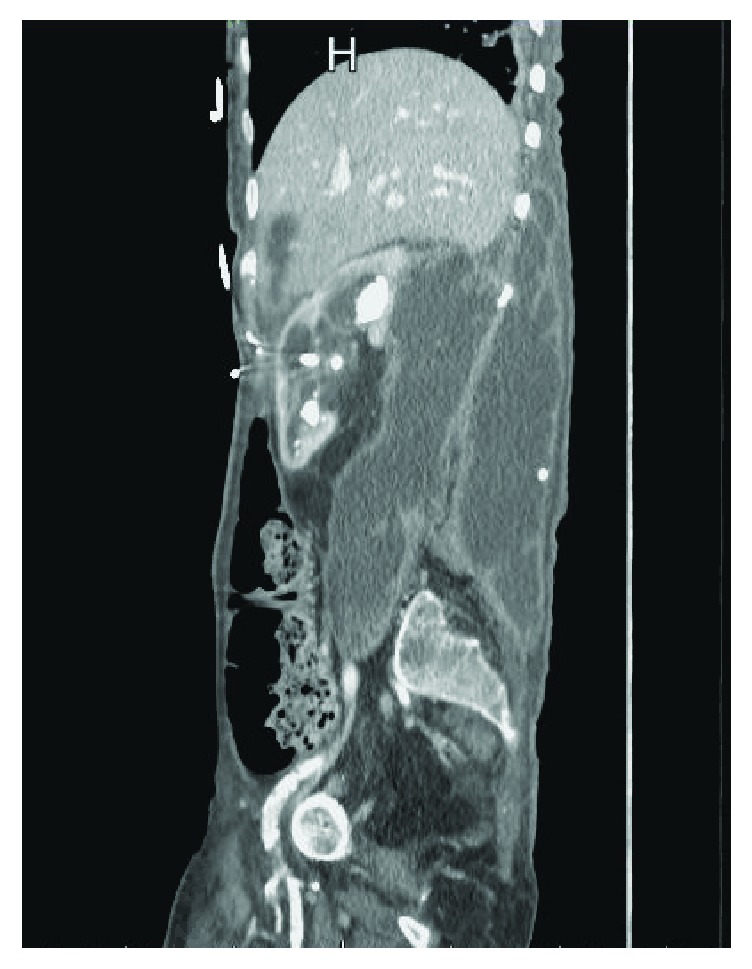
Sagittal section of a CT scan of the abdomen/pelvis with IV contrast revealing severe hydronephrotic right kidney with accompanying urinoma in the retroperitoneum.
